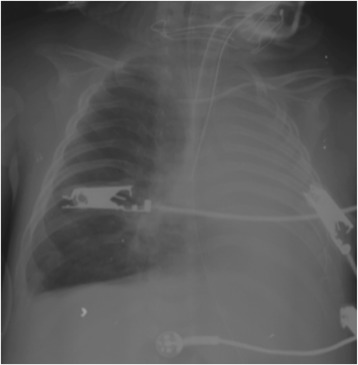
Chest radiographic image showing asymmetric lung injury with severe left-lung atelectasis and right-lung hyperinflation on the 14th day of hospitalization
